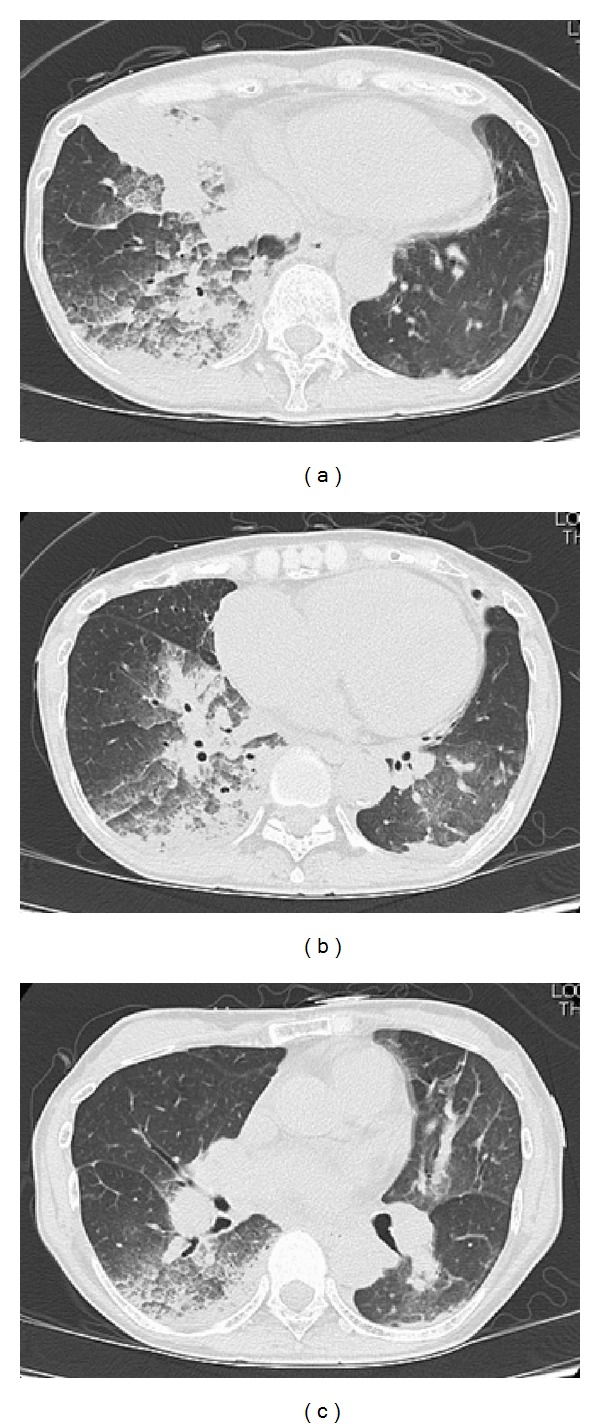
CT scan images obtained on the third postoperative day (POD3). The patient had localized consolidation on her right lower lobe with atelectasis, designating postsurgical pulmonary edema in the dilated segment.
